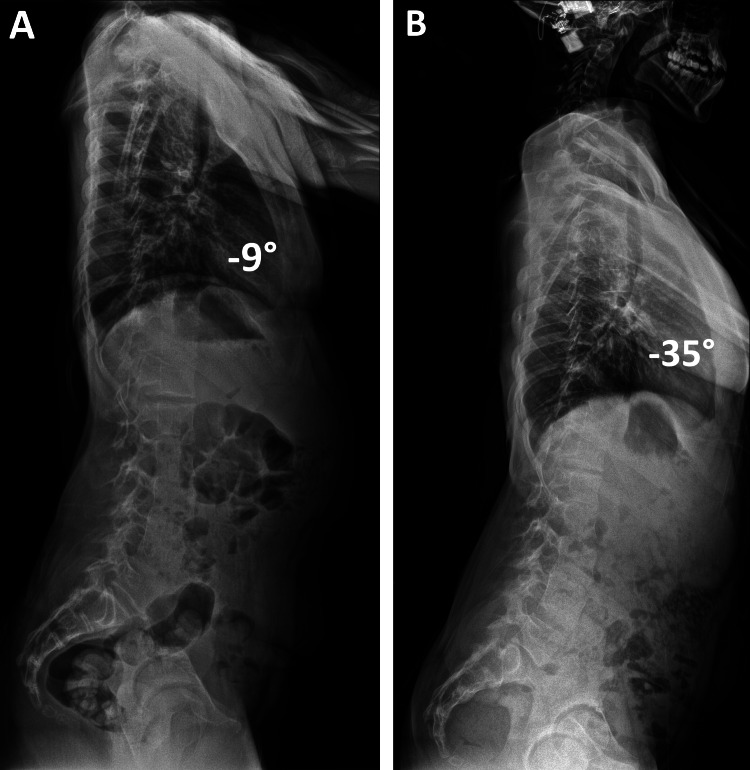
Radiographs showing the rapid progression of thoracic lordosis. Lateral radiographs of the spine taken at initial diagnosis in the patient's local service (A) and when she attended our clinic for the first time (B) show thoracic lordosis that deteriorated rapidly in a period of accelerated pubertal growth spurt, causing spinal penetration into the chest with consequent airway compression. The cochlear implant is noted in situ (B).
